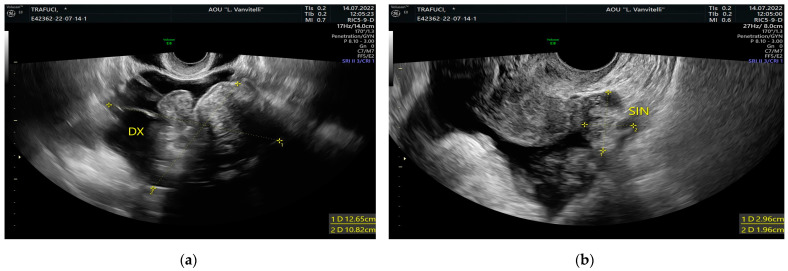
Transvaginal ultrasound image of adnexa: (a) right (e.g., *DX*) adnexal mass; (b) normal left (e.g., *SIN*) ovary.
